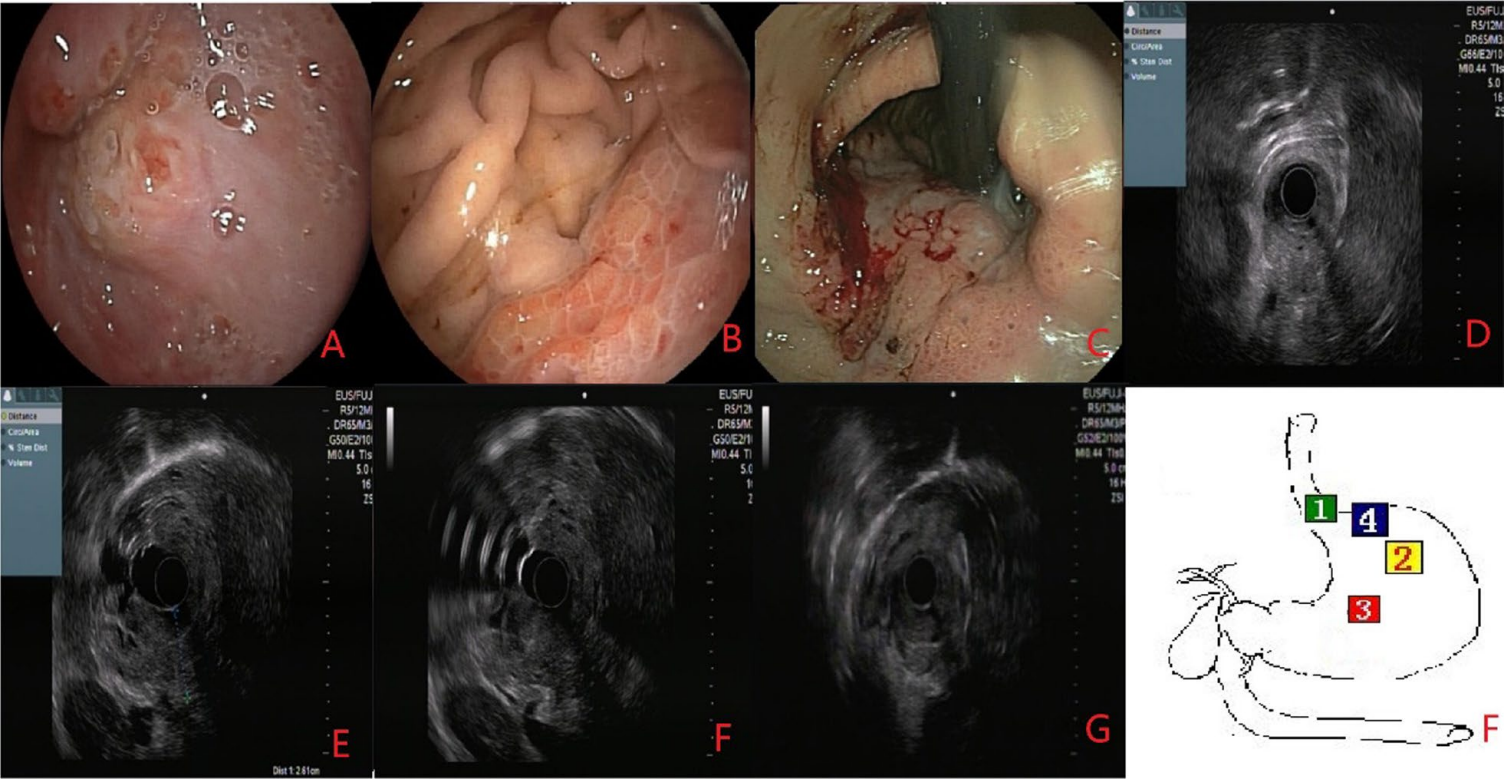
As shown in A, the mucosa at the SCJ was also partially congested and edematous. After the gastroscope passed through the cardia (B), it was evident that the lesion involved the fundus and greater curvature of the stomach. The diseased mucosa was congested, edematous, and bulged, resembling snakeskin. When we turned the gastroscope lens to observe the lesion closely (C), we observed the erosion and spontaneous bleeding. Endoscopic ultrasound showed thickened stomach wall, mucosal level disappearance, uneven echo of the lesion, manifested as low echo, and unclear boundary. The thickness of the thick part was about 2.6 cm, and the lesion invaded through the serosa, but we did not observe enlarged lymph nodes. The letters A–C in this figure correspond to the numbers 1–3 in F, and the letters D–G are all seen by ultrasound gastroscopy at the lesion, corresponding to the number 4 in F
